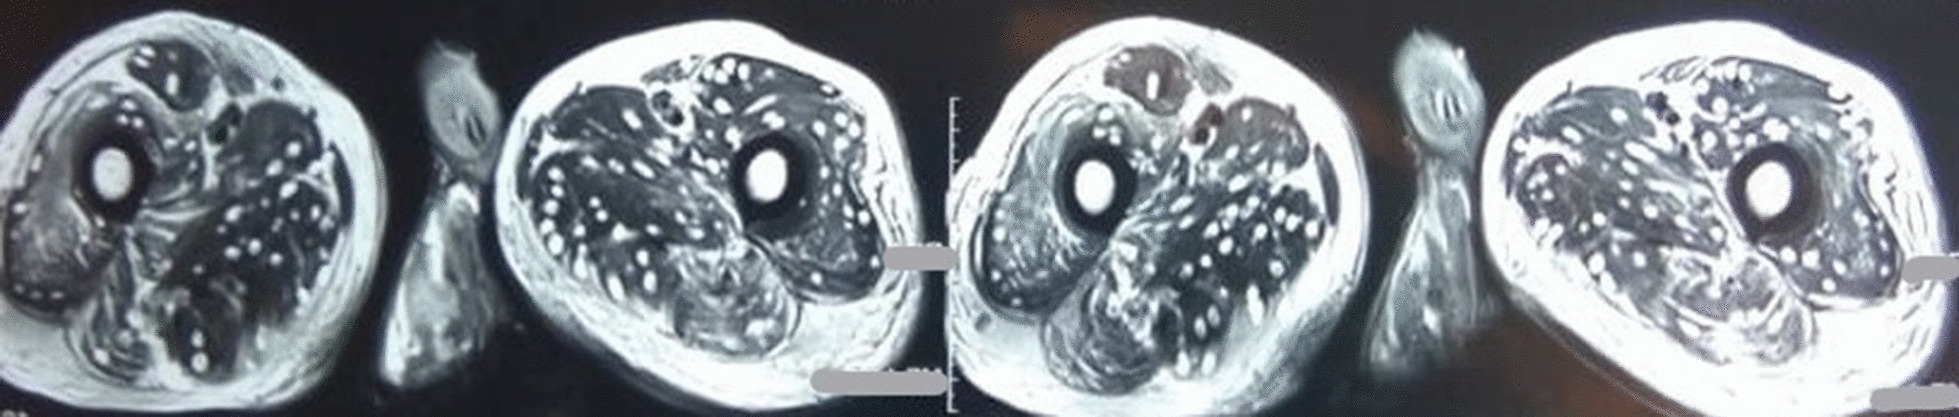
Magnetic Resonance Imaging of thigh muscles showing numerous cysticerci
